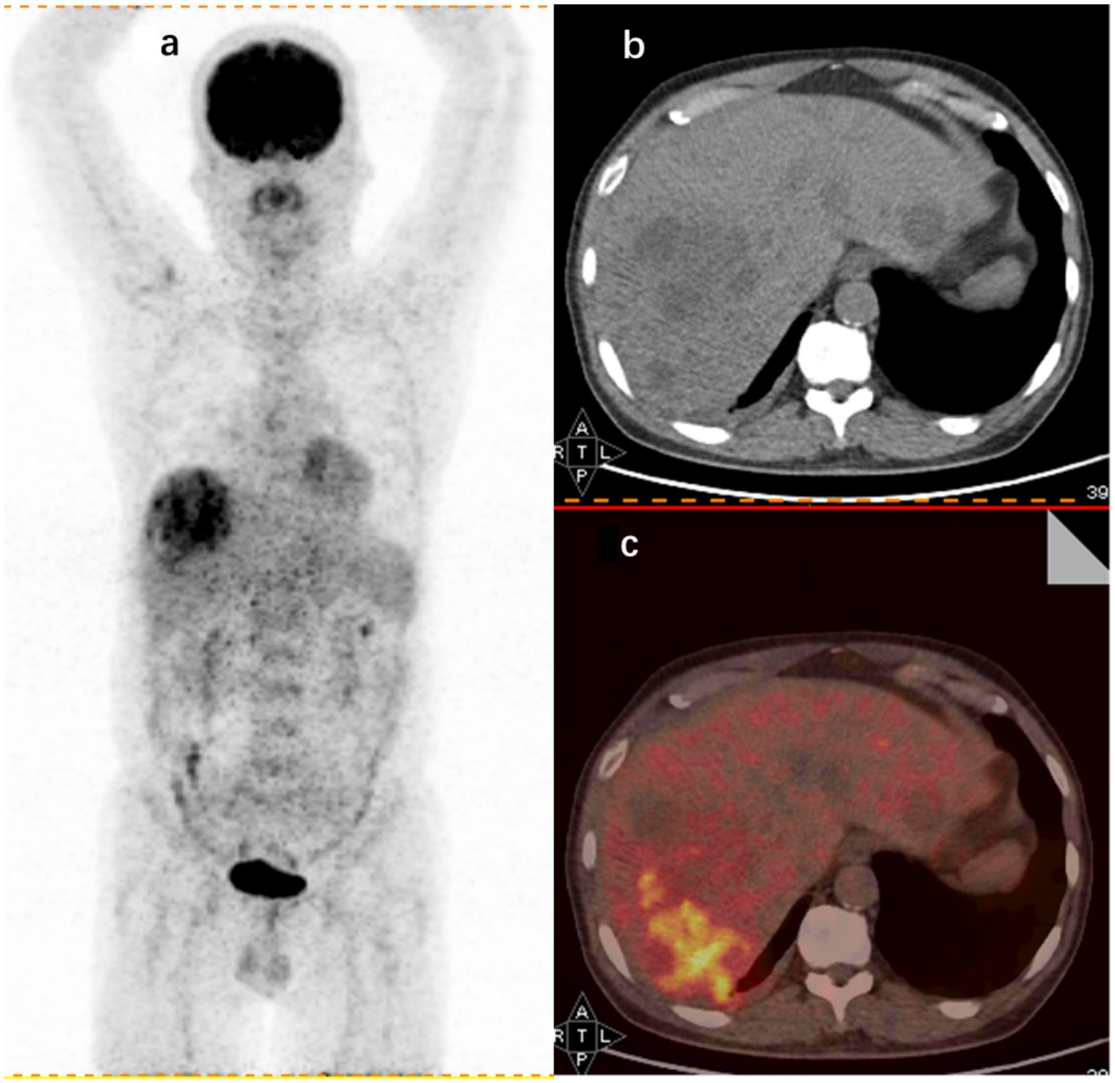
18F-FDG PET/CT findings in a 60-year-old male FUO patient with a final diagnosis of liver abscess. (A) Whole-body 18F-FDG PET coronal section showing an aggregated foci of metabolic activity in the right hepatic lobe. CT axial section (B) and imaging fusion (C) showing foci of increased uptake in the right hepatic lobe (SUVmax 8.1).
